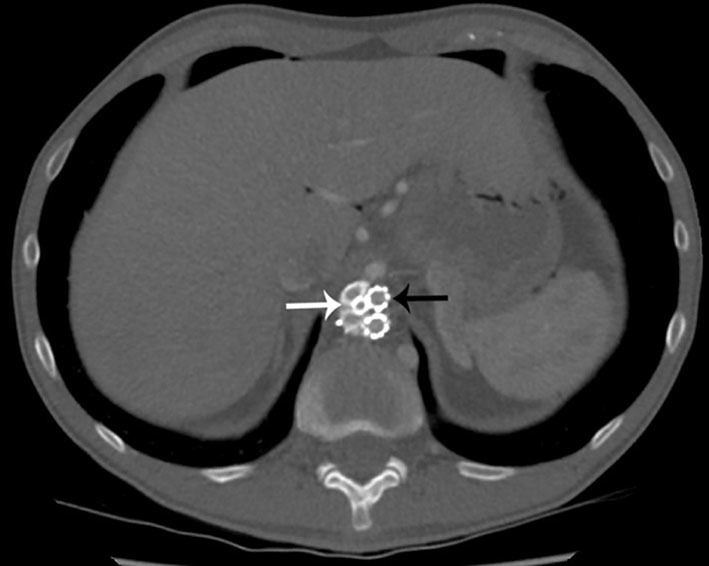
Axial CT image demonstrating the three-vessel chimney graft (black arrow) and the Nellix endograft (white arrow) within the abdominal aorta.
